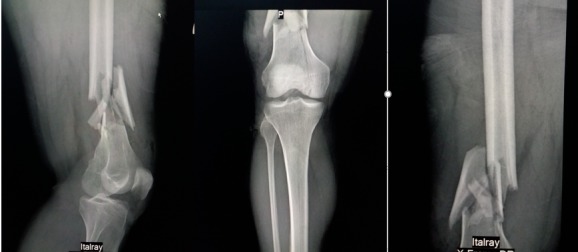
radiographie du genou face montrant une fracture supra condylienne avec un trait de refend sur le condyle médial, cependant sur le profil on note une ascension de la rotule
